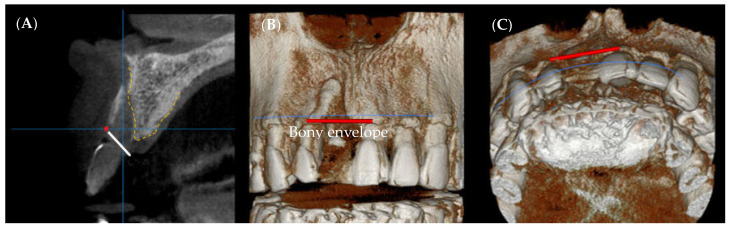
(A) CBCT sectional view. Note that the bony envelope appears as a red spot, (B,C) 3D radiographic analysis of bony envelope level.
